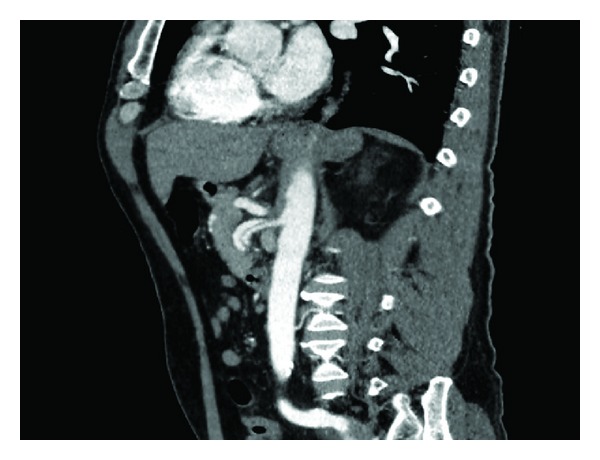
Computed tomography angiogram (sagittal view) depicting the dissection of the superior mesenteric artery extending from the orifice without involving any branch vessel.
